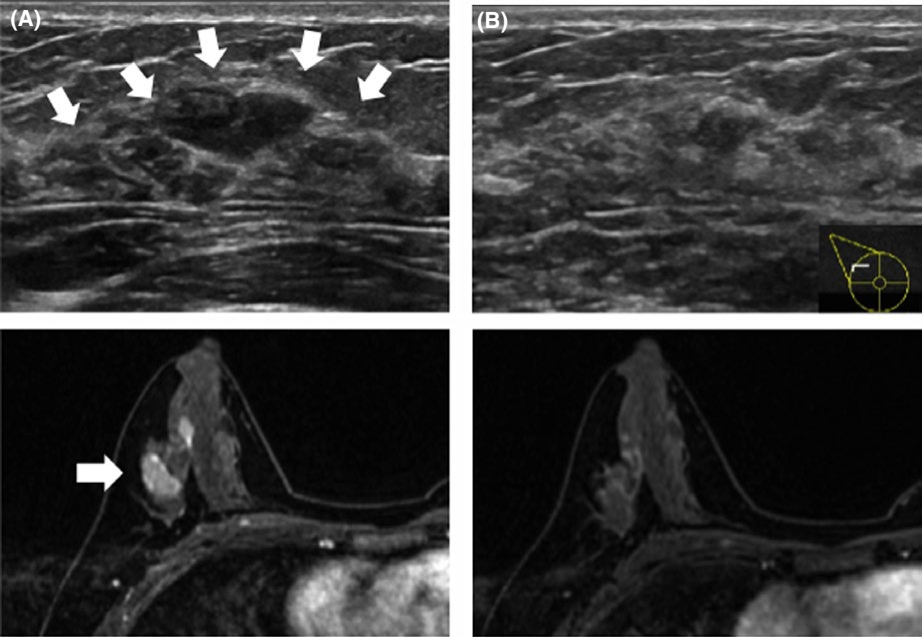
Ultrasonography and MRI of the right breast. A, Before treatment. Ultrasonography showed an irregularly shaped 20 mm low echoic breast mass in the outer‐upper quadrant. MRI noted an irregularly shaped 25 mm contrasted mass. The shape was irregular, the margin was not circumscribed, internal enhancement characteristics were heterogeneous, and kinetic analysis was rapid‐persistent. B, After treatment with 8 weeks of prednisolone. In the ultrasonogram, the right breast low echoic mass almost disappeared. MRI showed the right breast contrasted mass almost disappeared
